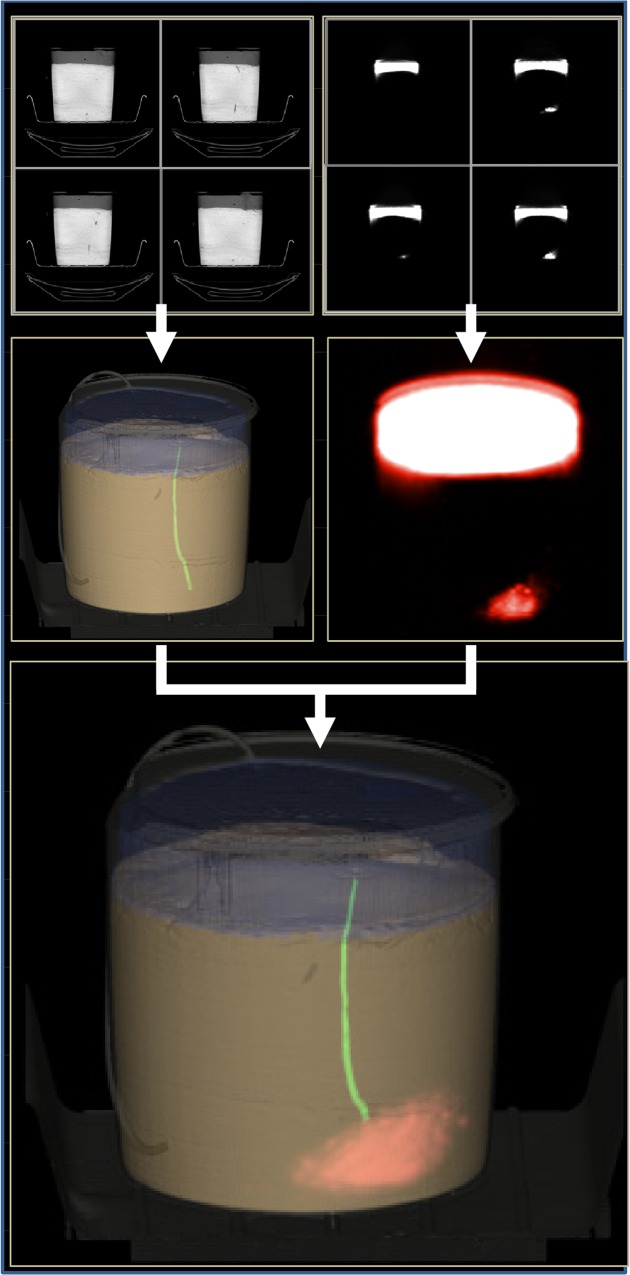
Construction of 3D images showing bioirrigating lugworm in sediment. A series of 2D cross-sections were assembled by computer to create full 3D images of the object (CT) and water injected with tracer (PET). Finally the co-registered 3D images are combined to form the resulting picture after 37 min. Artificial colors are used to highlights different components. The bucket was 25 cm in diameter.
